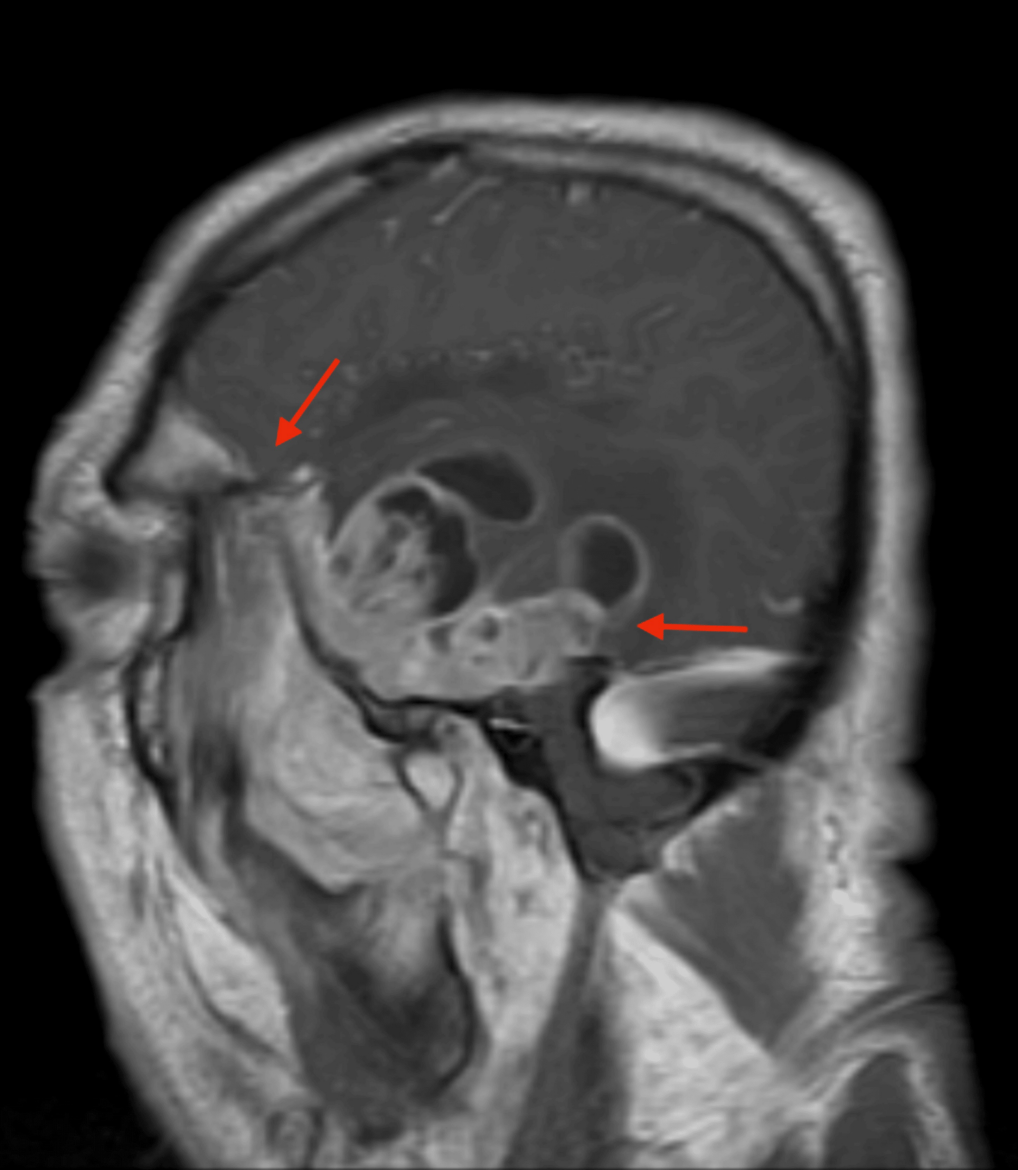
MRI of the brain MRI of the brain showed the right temporal lobe tumor extending into the sphenoid sinuses, the right cerebello-pontine angle, and the right retro-orbital region (red arrows).
